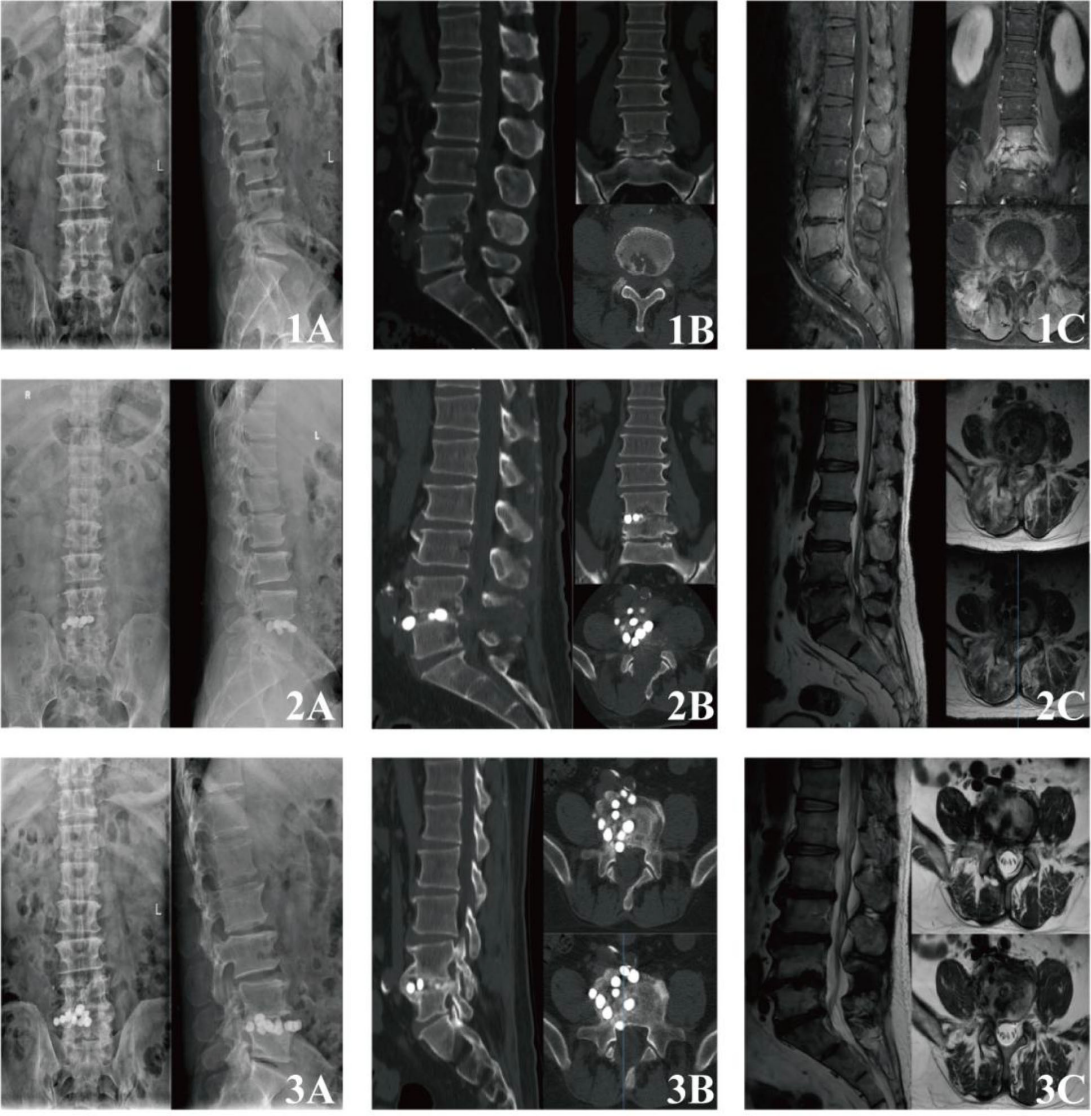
Preoperative and postoperative radiological imaging of typical cases: (1A-1C) Preoperative imaging. Radiography and CT demonstrate loss of disc height at L4–5, accompanied by endplate erosions and osteolytic destruction of the inferior endplate of L4 and superior endplate of L5. Contrast-enhanced MRI reveals diffuse enhancement of the L4 and L5 vertebral bodies, along with the posterosuperior portions of S1 and S2. The L4–5 disc shows irregular linear enhancement. An enhancing fluid collection (abscess) is observed within the spinal canal, extending cranially to the L2–3 level. (2A-2C) Immediate postoperative imaging. Radiography and CT confirm adequate positioning of radiopaque bone cement beads within the intervertebral space, which maintain distraction and restore segmental height. No significant bead fragmentation or migration is observed. MRI demonstrates marked regression of the pre-existing paraspinal and epidural abscesses. Bone marrow edema in the adjacent vertebrae is significantly reduced on T2-weighted sequences. The beads themselves appear as signal voids on all sequences. (3A-3C) Imaging at final follow-up. Radiography and CT show well-maintained vertebral and intervertebral height. Signs of osseous repair are evident at the previously infected sites, characterized by resolution of erosions with marginal sclerosis and new bone formation. There is no progressive bony destruction or vertebral collapse. Bone osteophytes have formed from the vertebral endplates, bridging the intervertebral space to form continuous bony bridges, indicating stable anterior column fusion. MRI confirms complete resolution of the previous vertebral bone marrow edema and paraspinal/epidural abscesses. The cement beads are encapsulated within a well-defined fibrous capsule.
